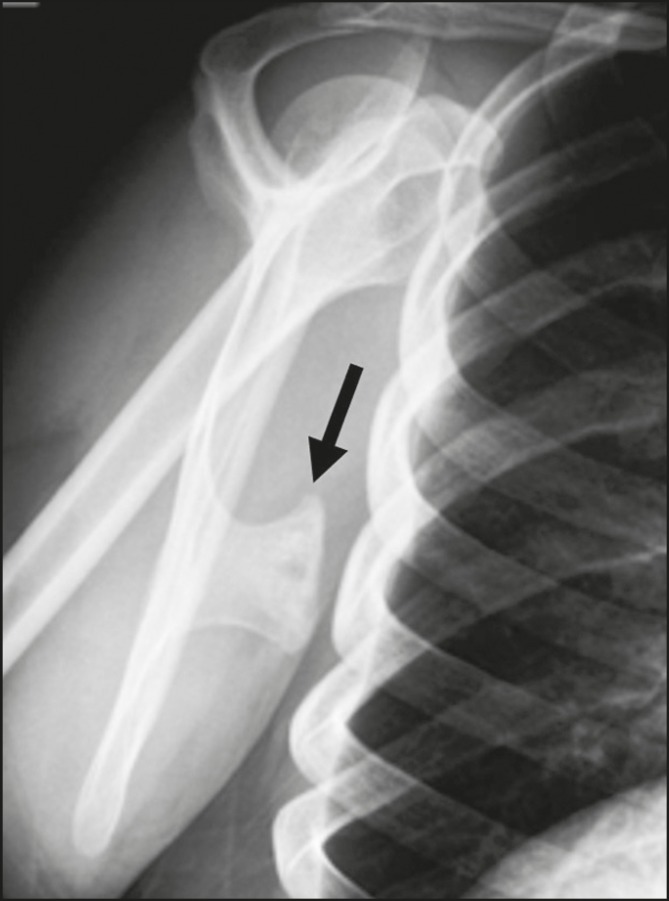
An 18-year-old male patient with pain in his right arm. X-ray showing
